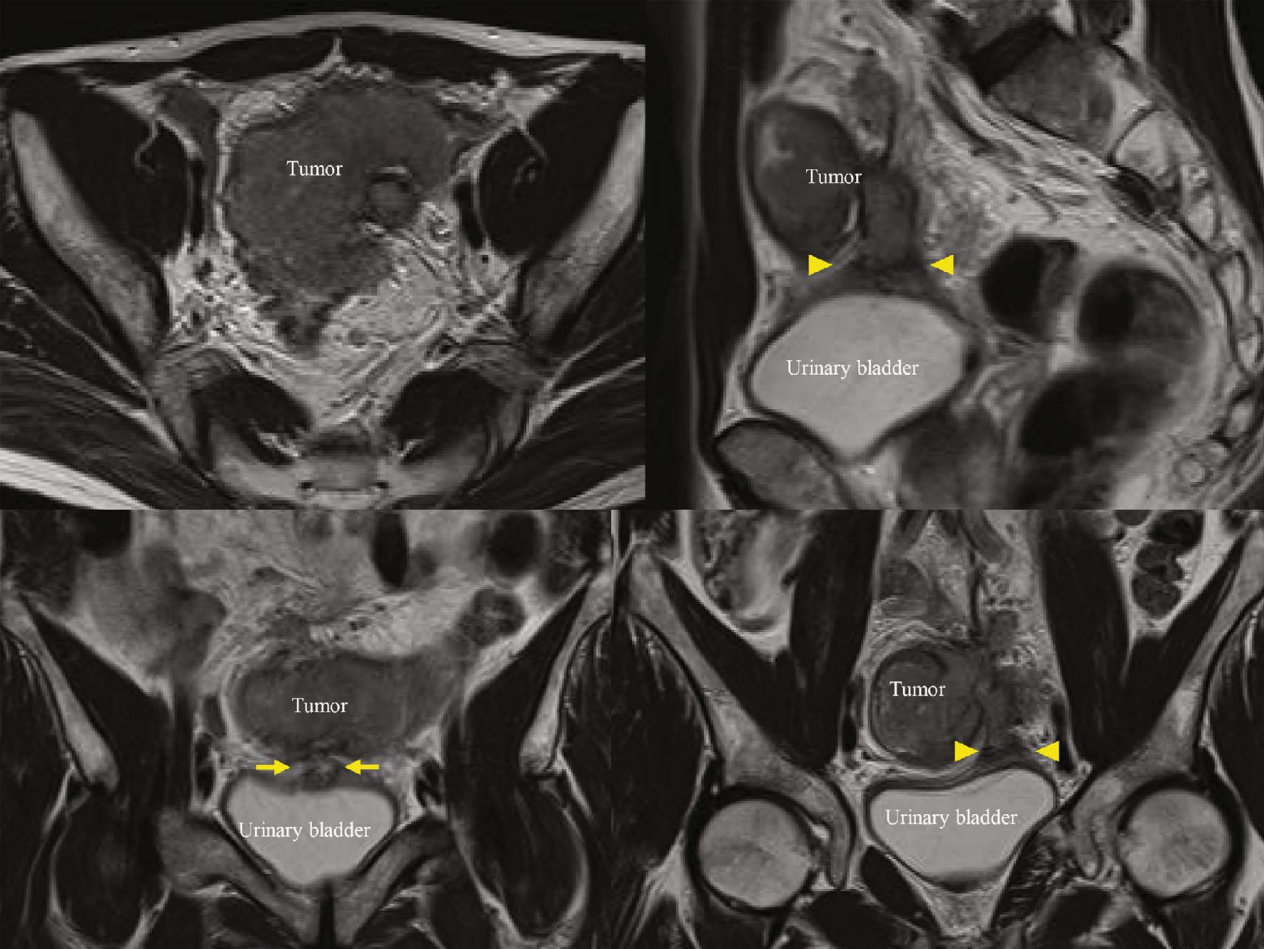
Magnetic resonance images show a large tumor directly invading the urinary bladder (arrowhead) and an abscess between the tumor and urinary bladder (arrow).
